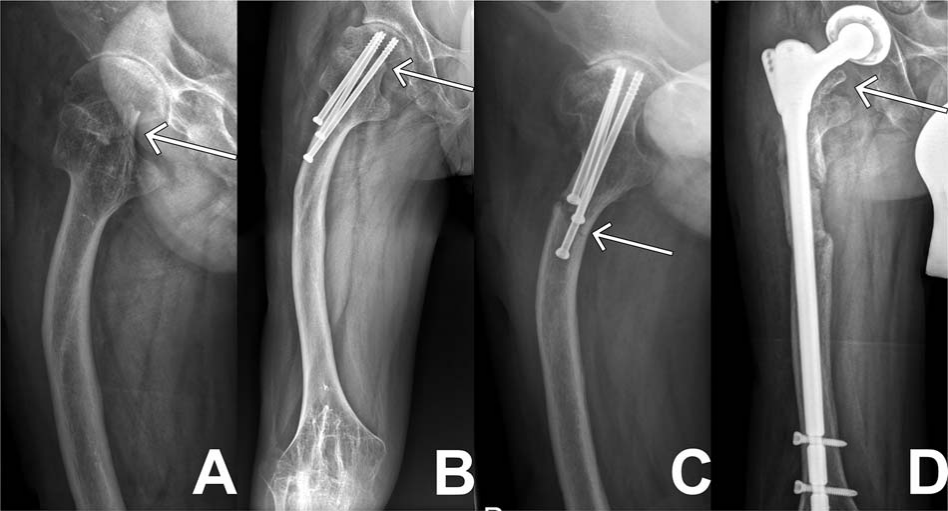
X-ray sequences of the right hip in different resolutions. (A) Representation of the medial femoral neck fracture before the first intervention as highlighted by arrow. (B) Osteosynthesis intervention of hip is highlighted by arrow few months after intervention. In addition, femoral deformity and knee rigidity are recognizable. (C) Periosteosynthetic fracture of the femoral shaft shortly before intervention. (D) Follow-up image of the inserted prosthesis material shows regular position.
